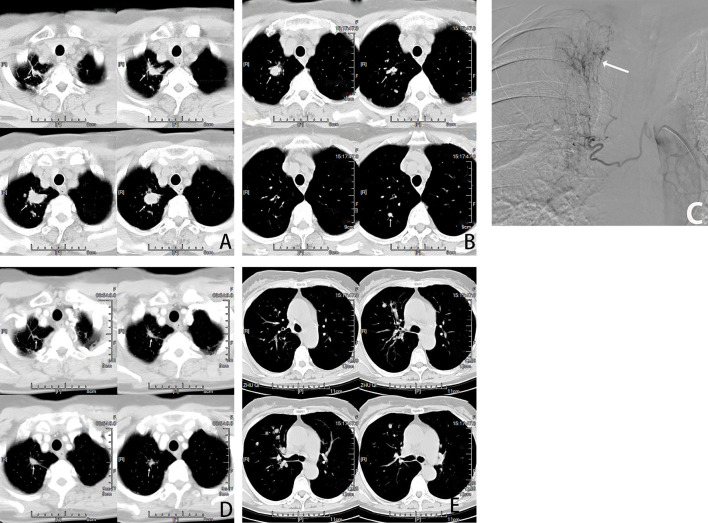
Female, 66 years old, lung adenocarcinoma treated with molecularly targeted drug therapy (gefitinib) for 2 years after tumor progression with multiple nodal metastases in the right lung. (A, B) are CT cross-sectional images of the patient, showing Multiple nodules(→) in the right lung. (C) is a right bronchial arteriogram showing that the right lung tumor(→) is supplied by the right bronchial artery. BACE chemotherapeutic agent: pemetrexed 800mg + carboplatin 300mg and gelatin sponge particles. (D, E) show that most of the nodules(→) in the lungs disappeared and shrunk after two courses of BACE, and PR was assessed.
